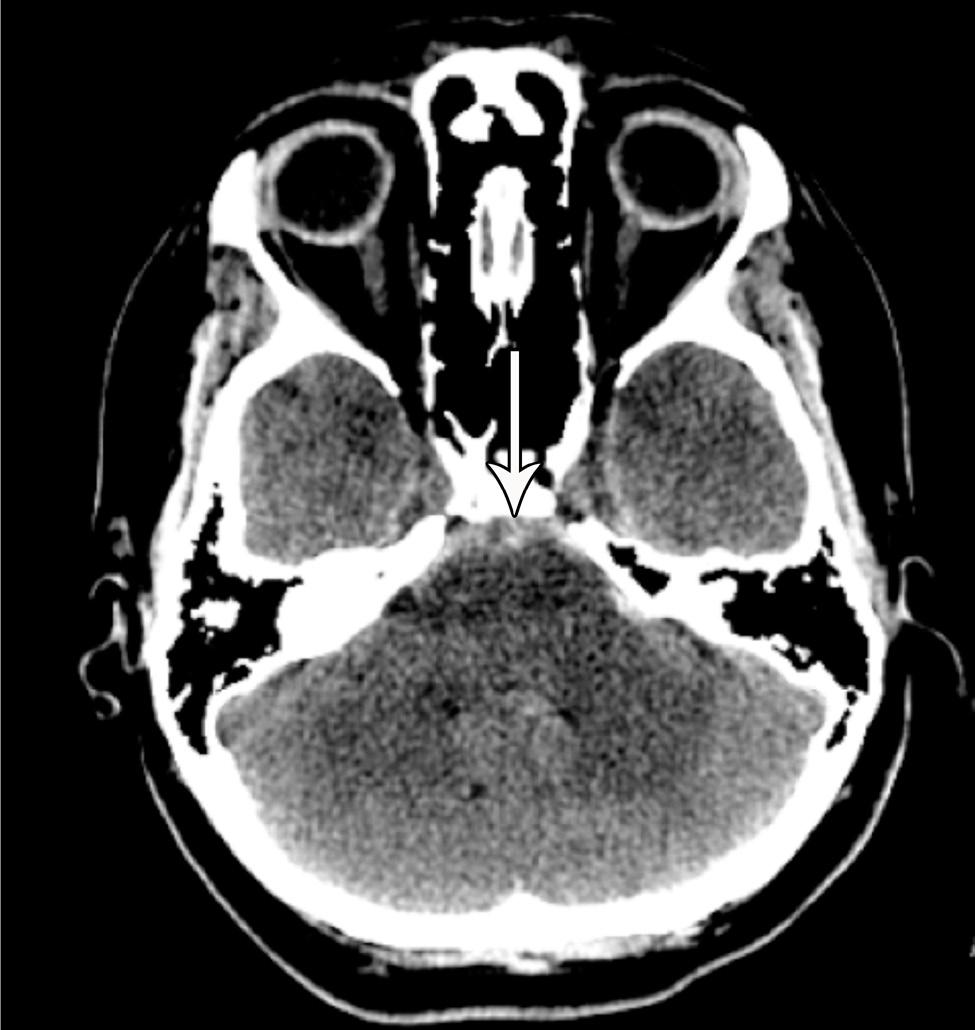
Head CT demonstrate subarachnoid hemorrhage anterior and lateral to the pons.
